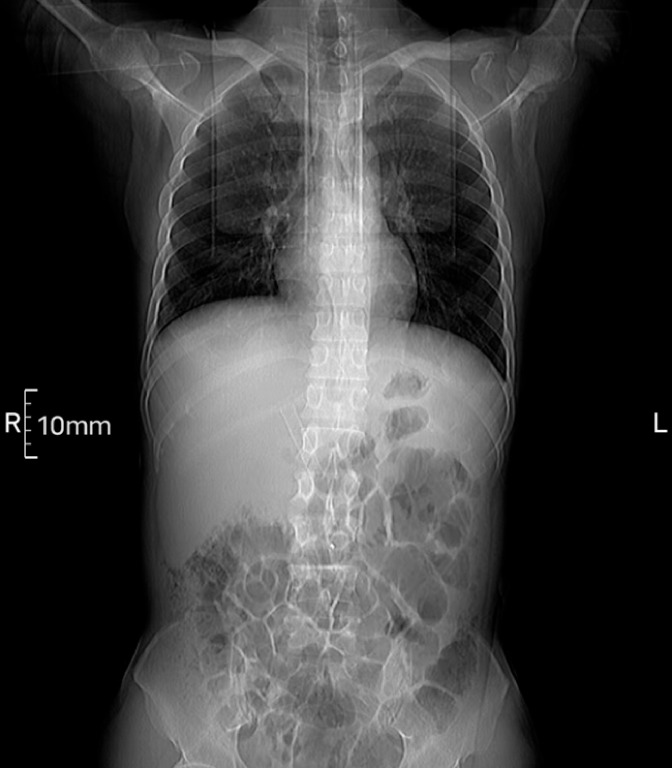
Abdominal coronal computed tomography showed migration of the biliary fully covered self-expandable metallic stent (FCSEMS).
